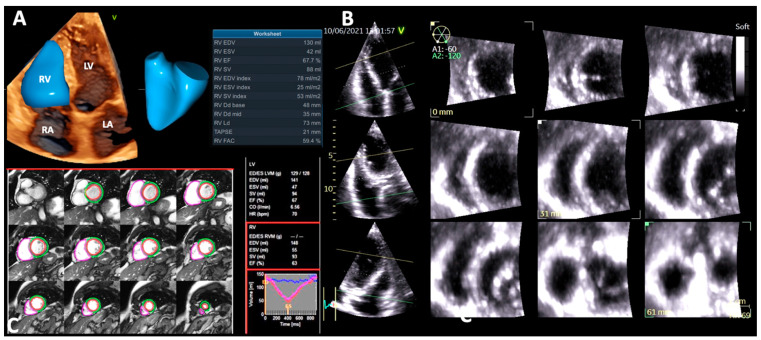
Right ventricular geometry and function in a normal subject assessed by three-dimensional echocardiography. (A) Right ventricular volumes and ejection fraction. (B) Multi-slice view of the right ventricle from the three-dimensional echocardiography data set showing the crescent shape in cross-section and triangular shape when viewed from the side. (C) Right ventricular geometry and function in a normal subject by cardiac magnetic resonance. Abbreviations: CO, cardiac output; Dd, diastolic diameter; EDV, end-diastolic volume; EF, ejection fraction; ESV, end-systolic volume; FAC, fractional area change; HR, heart rate; LA, left atrium; LD, longitudinal diameter; LV, left ventricle; LVM, left ventricular mass; RA, right atrium; RV, right ventricle; RVM, right ventricular mass; SV, stroke volume; TAPSE, tricuspid annulus plane systolic excursion.
